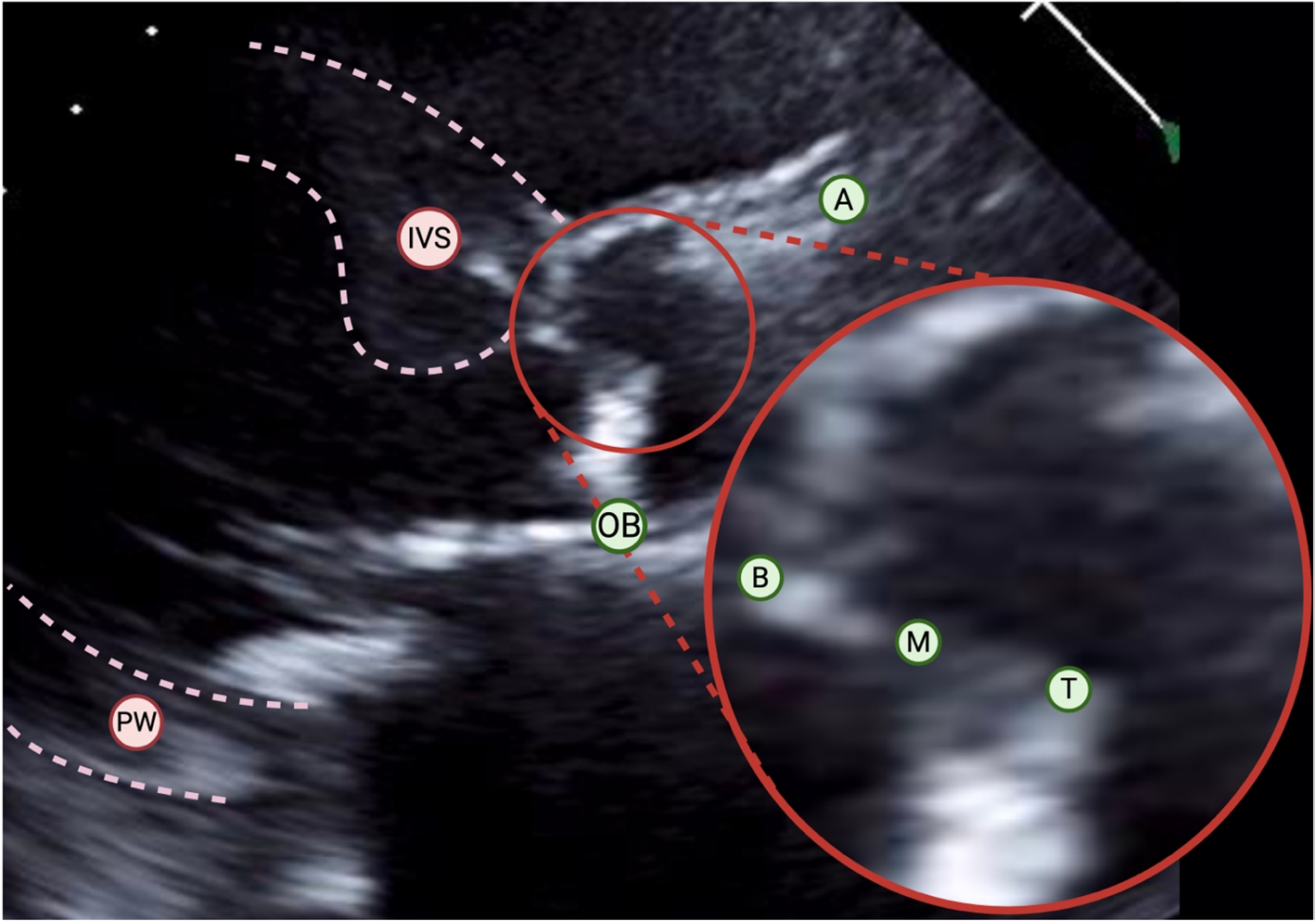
Parasternal long axis image with annotated landmarks. Three landmarks were created along the anterior (right coronary) leaflet (inset), in addition to the aortic wall and opposing leaflet hinge point, to calculate vectors. These vectors are then tracked in systole and diastole to generate motion data. A, aortic wall; B, base; IVS, interventricular septum; M, midpoint; OB, opposing base; PW, posterior wall; T, tip
